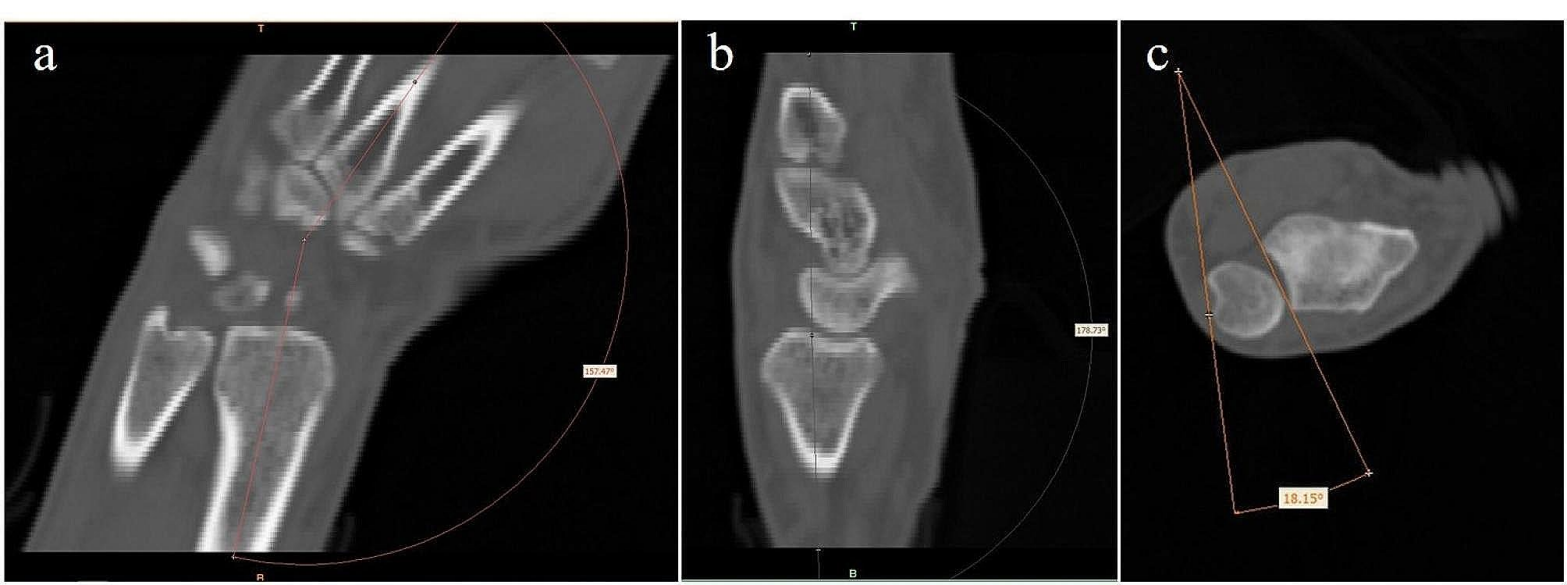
In the CT images, longitudinal axes of the third metacarpus and of the distal radius in the coronal plane and in the sagittal plane forming the angles of the radio-ulnar deviation (a) and the flexion-extension (b), respectively, and lines connecting two tips of the radial sigmoid notch and of the ulnar ECU (extensor carpi ulnaris tendon) notch in the horizontal plane forming the angles of rotation (c)
